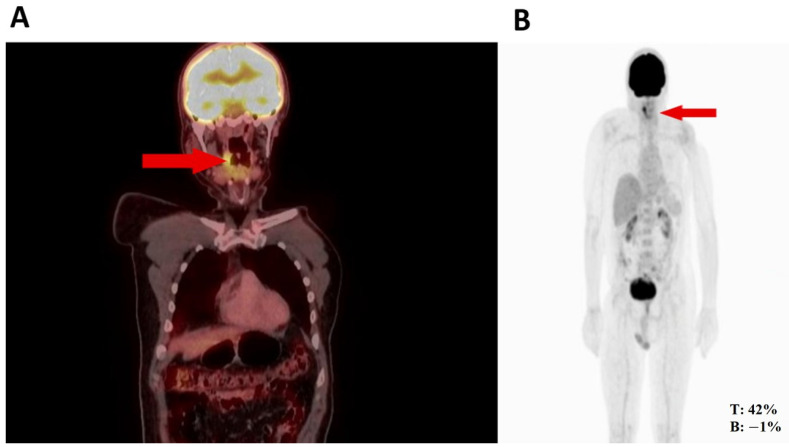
(A) Nodule in the right pharynx and palatoglossal arch measuring 22 × 10 mm, SUV 6.5 (red arrow). (B) Hypermetabolic nodular thickening of the right lateral pharyngeal wall, nonspecific, suspicious of neoplastic involvement (red arrows).
